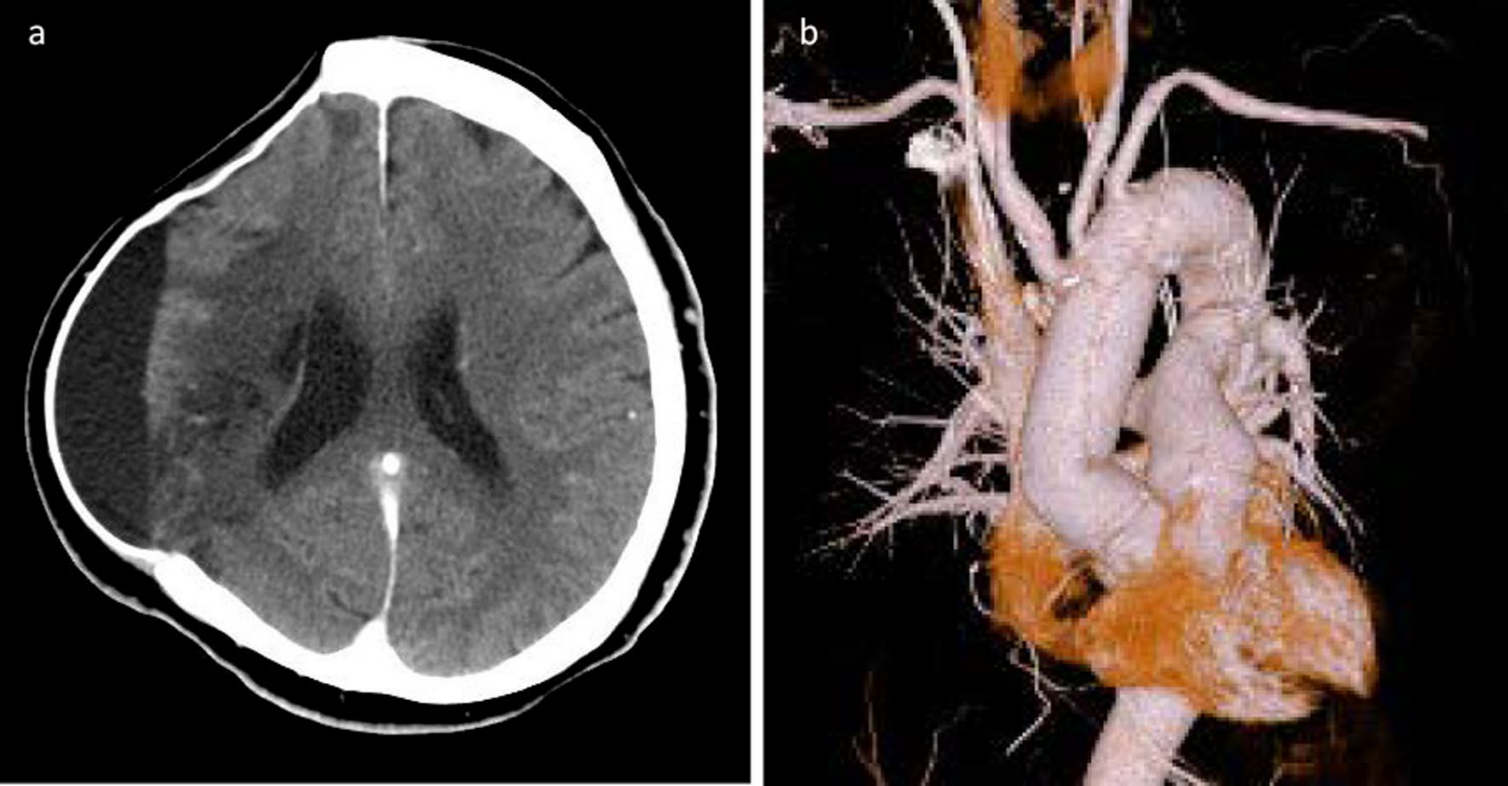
Postoperative CT shows the graft-replaced ascending aorta (a) and a moderate amount of cerebral spinal fluid (b)
